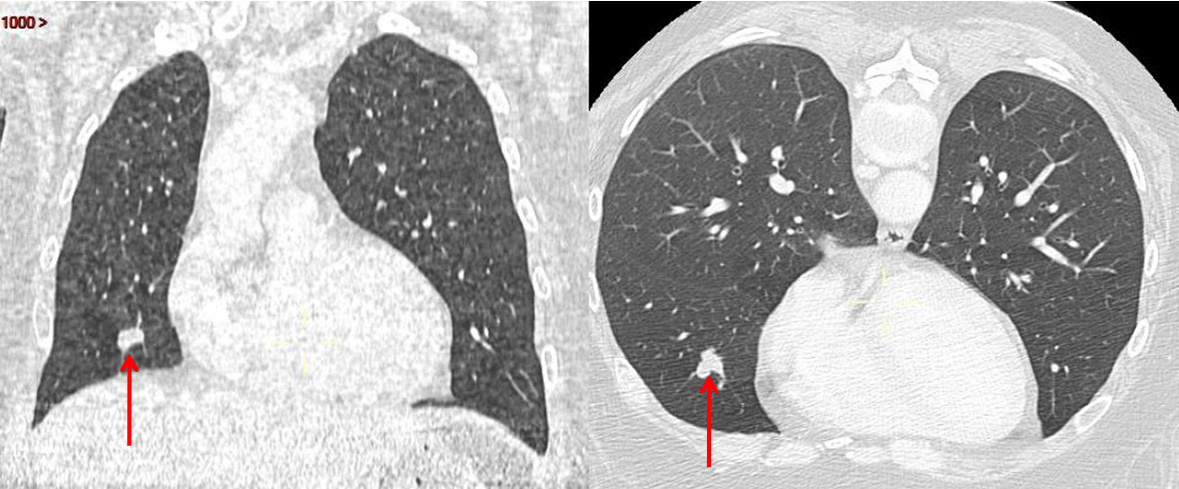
Frontal and axial chest computed tomography scans showing adenoid cystic carcinoma metastasis (red arrow) in the right middle lobe of the lung.
